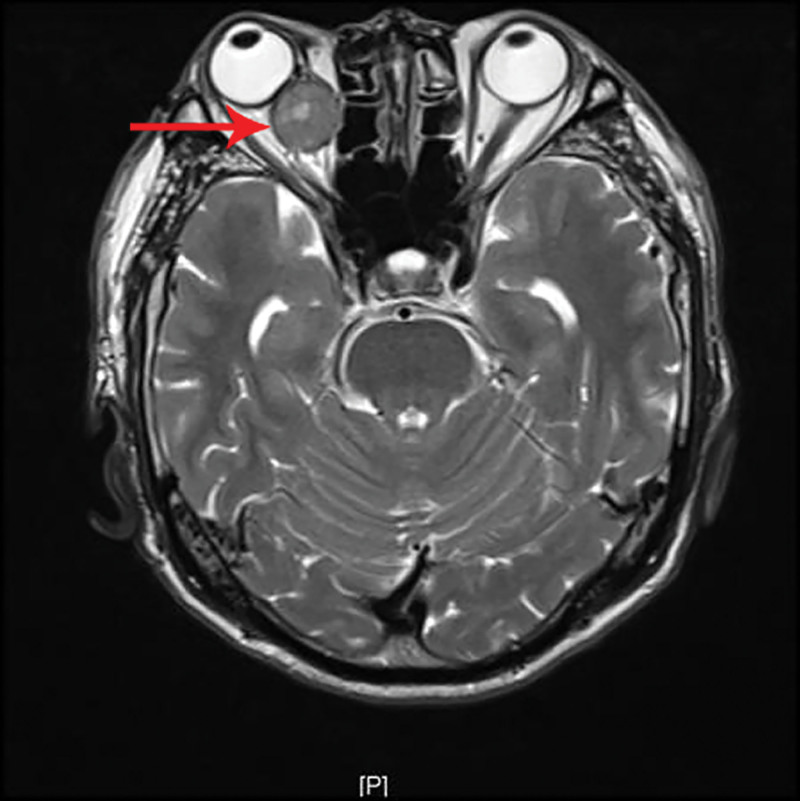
T2-weighted magnetic resonance imaging showing a retrobulbar mass with focal intralesional hyperintensity (arrow).
